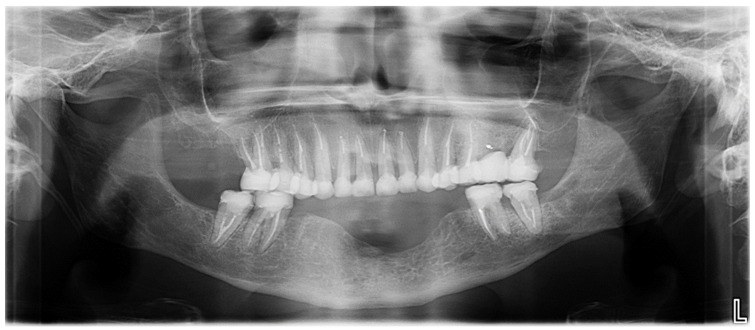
Panoramic radiograph after treatment.
